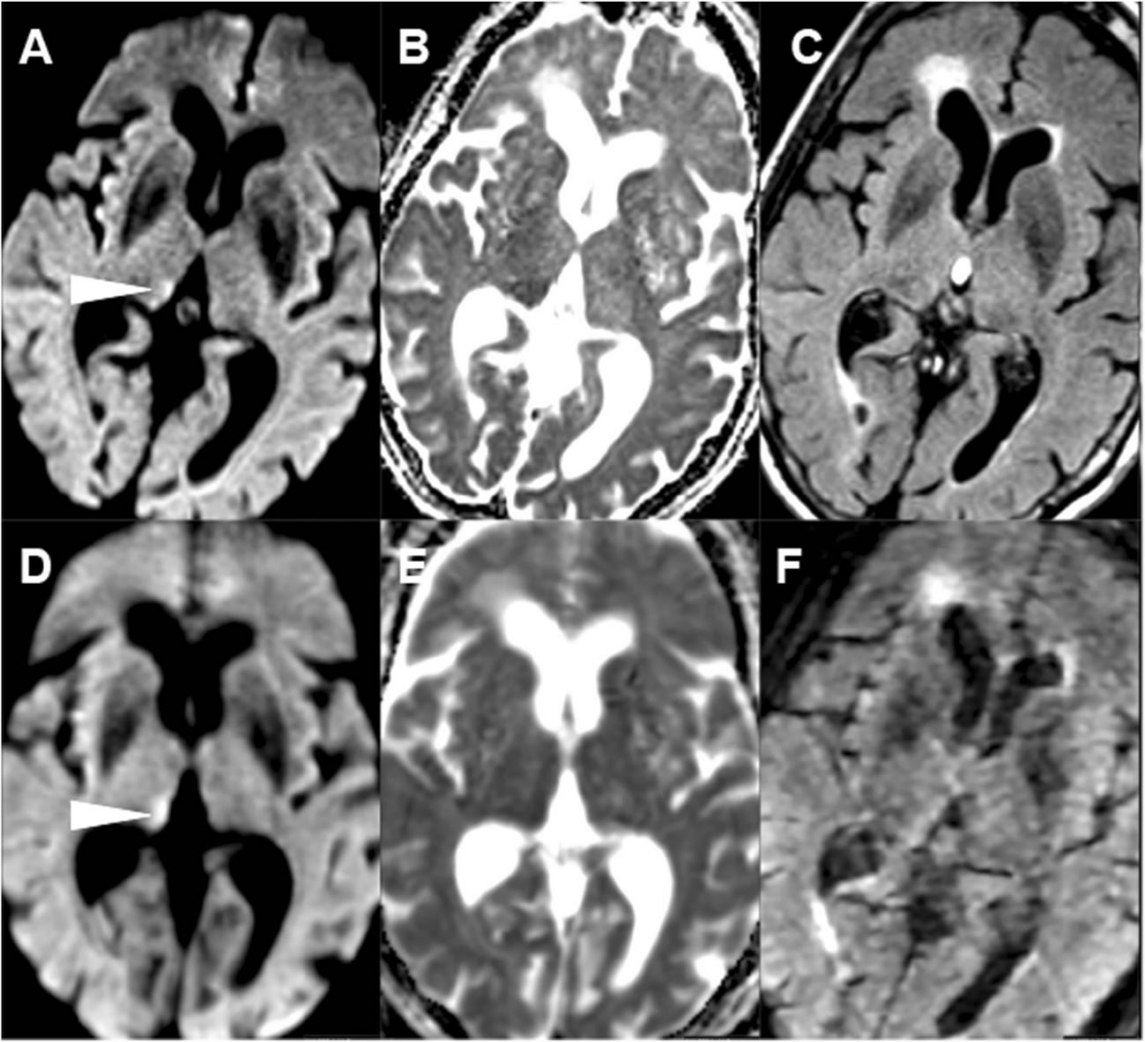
Images in an 80-year-old woman with left-sided weakness (NIHSS 24) and unknown onset of symptoms. CT without infarction or arterial occlusion on CT angiography. MRI was performed 1 h after CT to confirm infarction diagnosis. Axial DWI, ADC, and T2-FLAIR images, A–C NeuroMix upper row and D–F cMRI lower row. The patient could not lie still, resulting in motion artifacts, mainly on F cMRI T2-FLAIR and to a lower extent on D cMRI DWI. A, D A faint high-signal lesion is visible on DWI in the medial thalamus on the right side on both NeuroMix and cMRI (arrowhead). The lesion was classified as acute infarction by consensus reading. The lesion is not visible on NeuroMix T2-FLAIR, and cMRI T2-FLAIR was impossible to analyze due to motion artifacts. Notably, the clinical radiological reading detected no lesion with the interpretation that no infarction was present, reperfusion treatment was not administered, and the evolution of NIHSS was not documented
